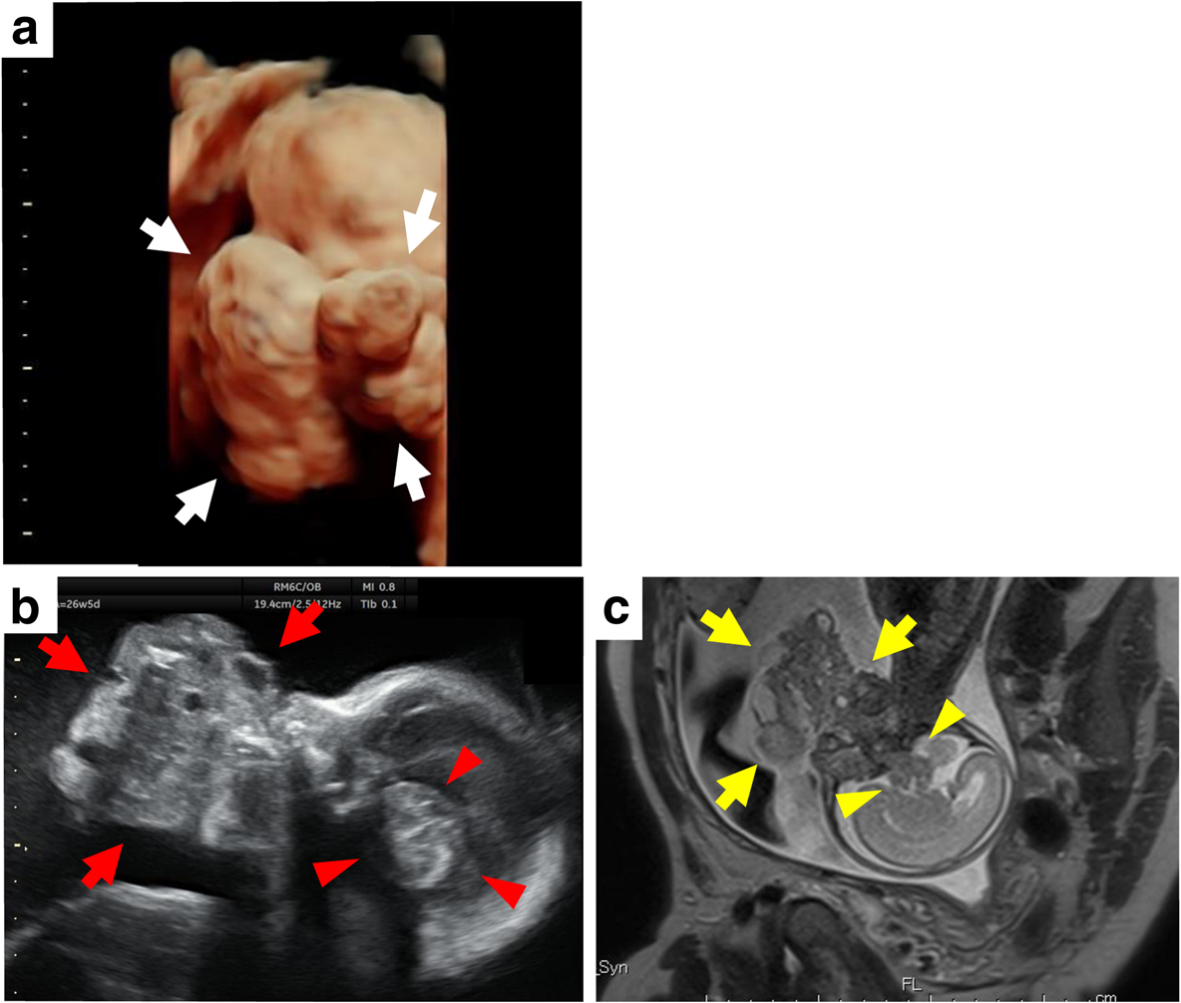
Ultrasonographic and MRI images. a Three-dimensional ultrasonographic image at gestational week 25 shows a giant tumor around the neck and face (white arrow), strongly suggesting an epignathus. b Ultrasonographic image at gestational week 26 shows a giant tumor around the neck (red arrow), and an intracranial tumorous lesion (red arrowhead). c MRI image at gestational week 25 suggests a connection between the giant tumor around the neck (yellow arrow) and the intracranial tumor (yellow arrowhead)
